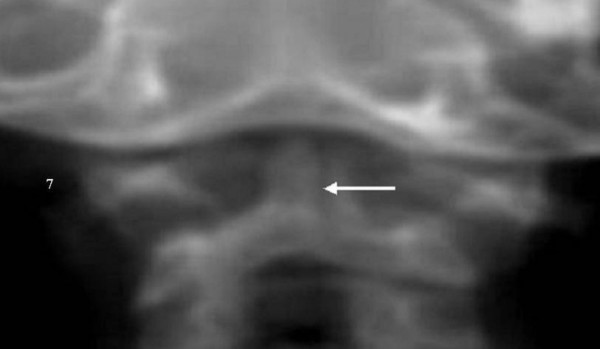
Coronal axial CT scan: Note the abnormal ossification and persistent radiolucencies of the two-paramedian columns. The arrow indicates an occult vertical fracture through the odontoid process and body of the axis.
